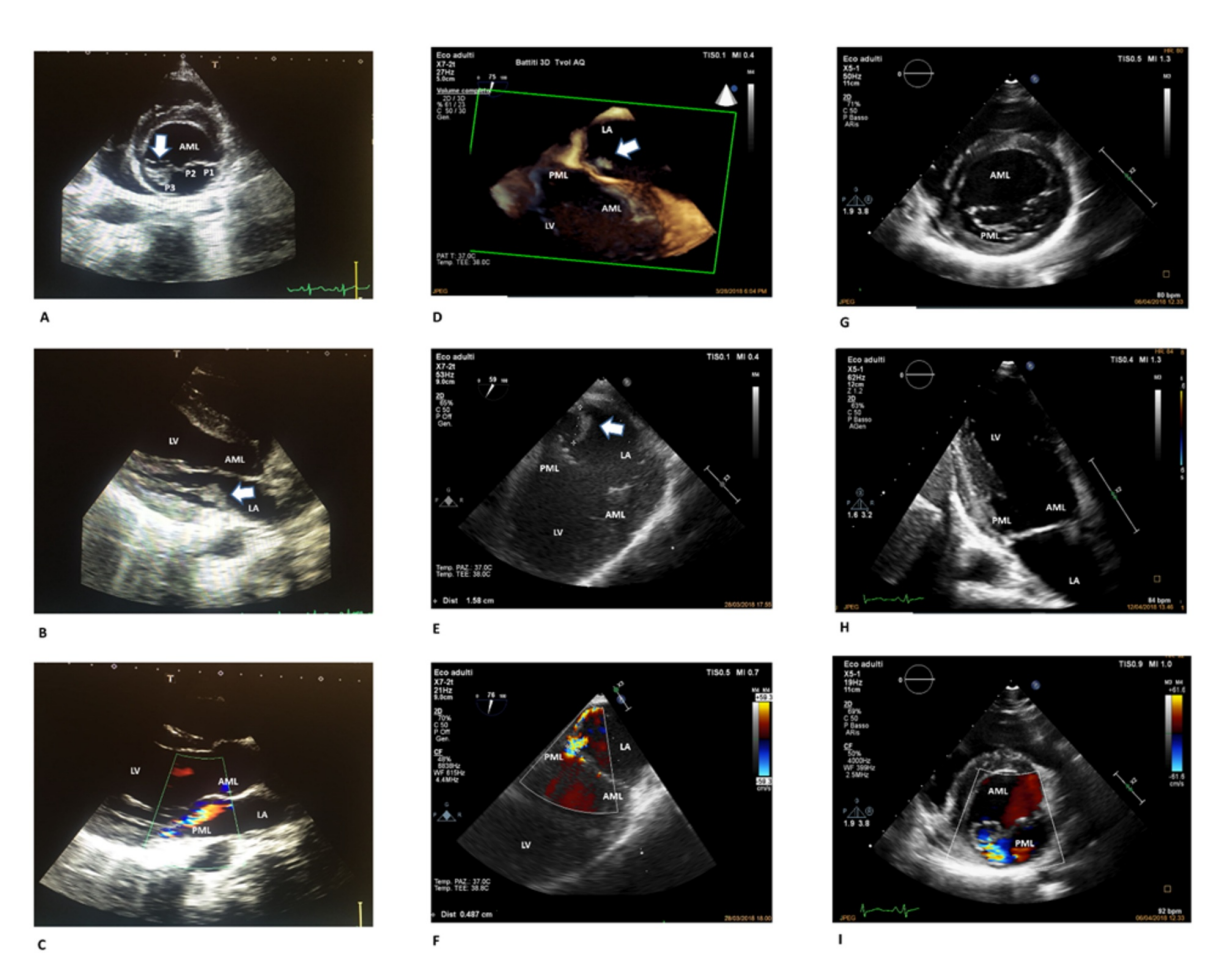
Sequential echocardiographic assessments Panel A: Transthoracic two-dimensional echocardiography – parasternal short axis view of the mitral valve. Arrow showing vegetation on the medial portion of the posterior mitral leaflet (P3). Panel B: Transthoracic two-dimensional echocardiography – parasternal long axis view. Arrow showing vegetation on the posterior mitral leaflet. Panel C: Transthoracic two-dimensional echocardiography – parasternal long axis view with color flow mapping displaying a mild mitral valve regurgitation. Panel D: Three-dimensional transesophageal view of the mitral valve. Arrow showing vegetation on the posterior mitral leaflet. Panel E: Transesophageal off-axis view of the mitral valve. Arrow showing vegetation on the posterior mitral leaflet. Panel F: Transesophageal off-axis view of the mitral valve with color flow mapping showing a moderate mitral regurgitation (vena contracta width 4.87 mm). Panel G: Transthoracic two-dimensional echocardiography – parasternal short axis view of the mitral valve. Resolution of the vegetation that was detected at the P3 level. Panel H: Transthoracic two-dimensional echocardiography – apical two chamber view. Anterior and posterior mitral leaflets appear intact without vegetation. Panel I: Transthoracic two-dimensional echocardiography – parasternal short axis view of the mitral valve with color flow mapping showing mild residual mitral regurgitation. Abbreviations: AML, anterior mitral leaflet; LA, left atrium; LV, left ventricle; PML, posterior mitral leaflet. P1 (lateral), P2 (middle), and P3 (medial) are the scallops of the posterior mitral leaflet.
